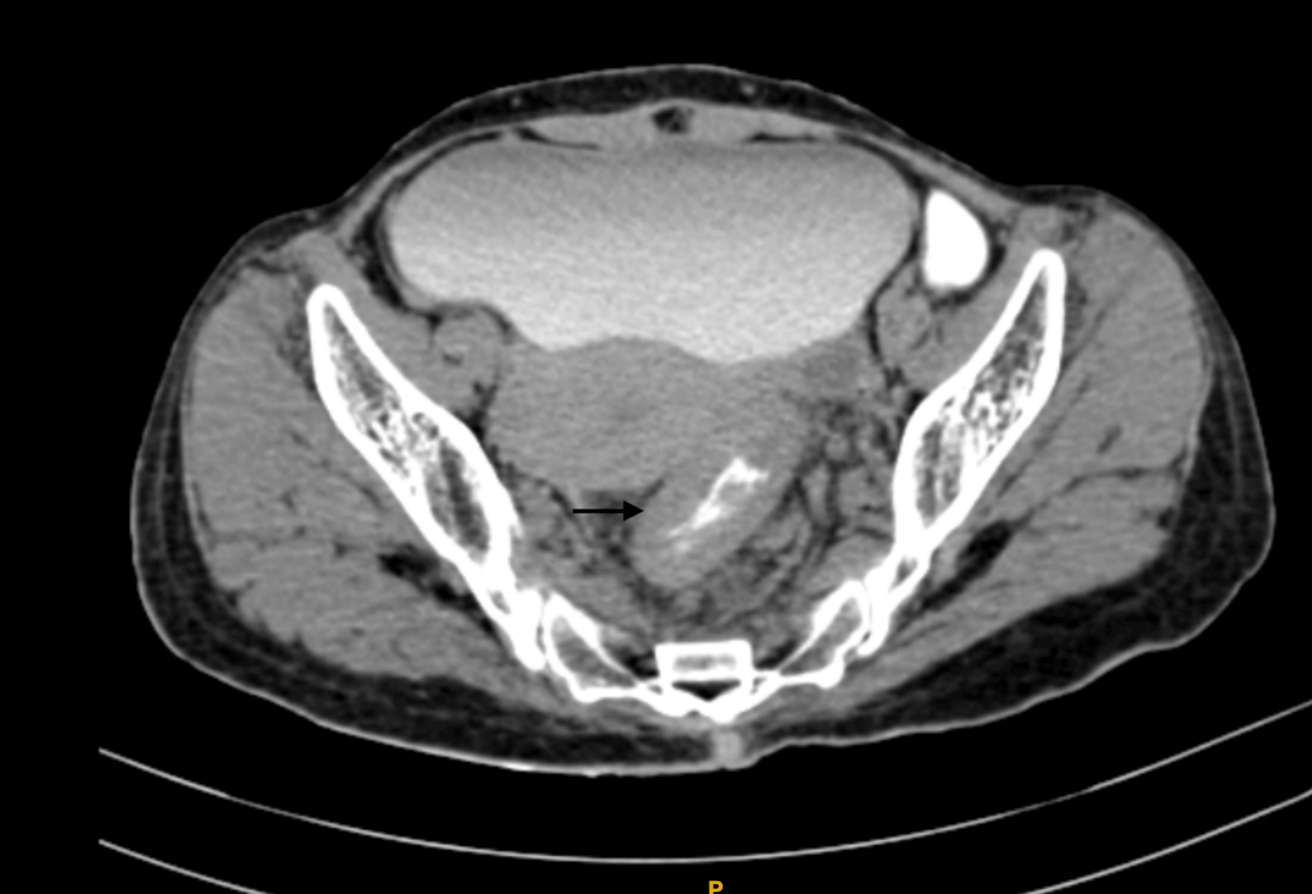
Abdominopelvic CECT scan findings The black arrow shows irregular circumferential wall thickening in the rectum, involving the rectosigmoid junction causing luminal narrowing. CECT: Contrast-enhanced computed tomography
